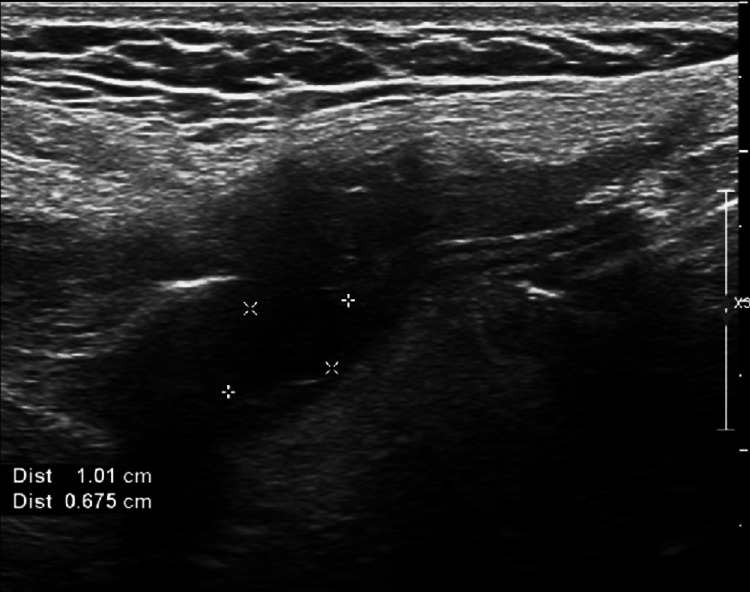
Ultrasonography of neck mass (longitudinal view).
